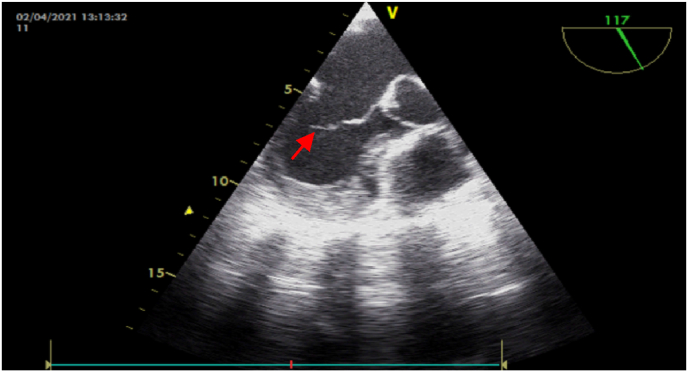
A transoesophageal echocardiography section showing vegetation at the anterior mitral valve.
